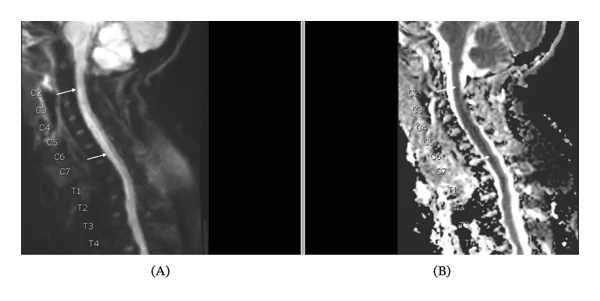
Sagittal DWI (A) and ADC (B) image of the cervical spine demonstrating high DW signal intensity involving the anterior cervical spinal cord (white arrows in A) appearing dark on ADC (white arrows in B) in keeping with an acute infarct.
